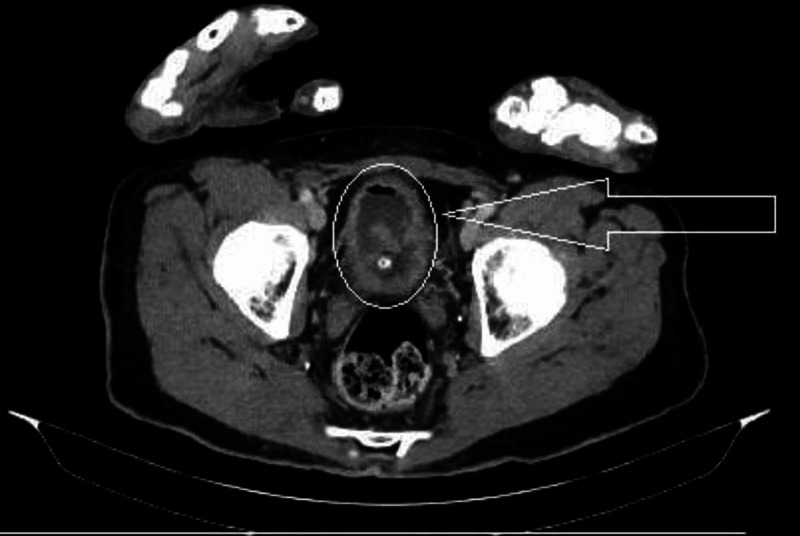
CT of abdomen-pelvis showed thickened bladder wall and polypoid projections into the bladder’s lumen
